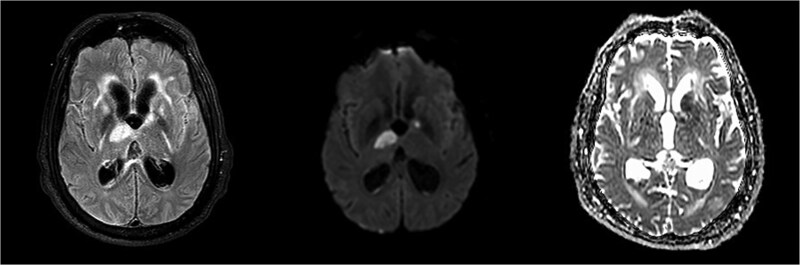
Repeat brain MRI showing additional acute infarcts in the right thalamus and left basal ganglia.
